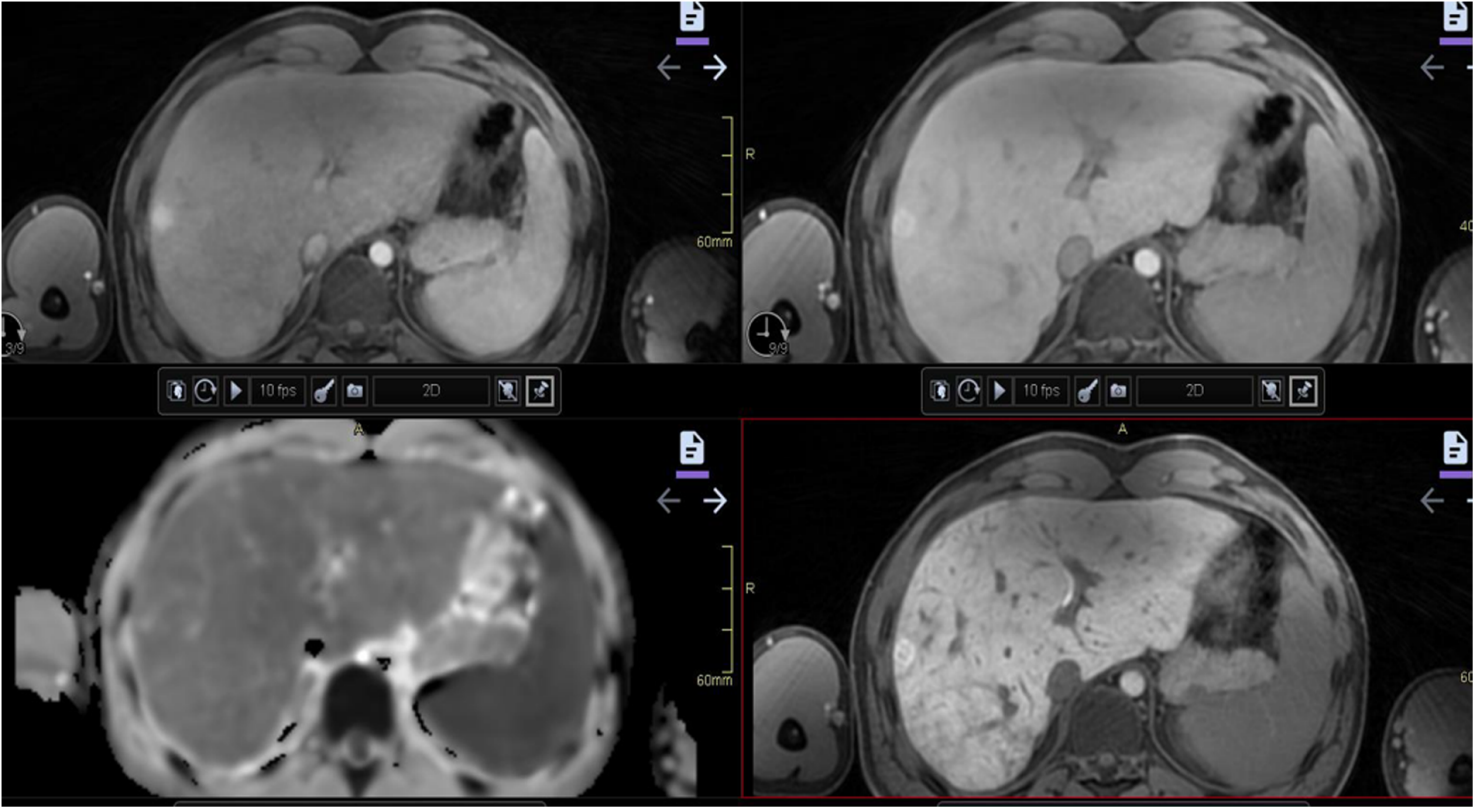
An 18-year-old boy born with double inlet left ventricle and pulmonary stenosis with a Fontan circulation. Satisfactory ventricular function was observed. Abdominal MRI was performed as a regular follow-up before transition to adult care. A 14 mm nodule not visible on ultrasound was found in the lateral part of the right liver lobe on MRI. Dynamic contrast-enhanced MRI with hepatocyte specific contrast agent was performed, revealing hyper-enhancement in arterial phase (upper left) and in late portal venous phase (upper right) as well as slightly irregular in hepatocyte phase (after 20 min) (lower right). The nodule is difficult to see clearly on the diffusion-weighted image (lower left). The nodule was interpreted with features of a probably benign nodule, and close follow-up with contrast-enhanced MRI was recommended. MRI, magnetic resonance imaging.
